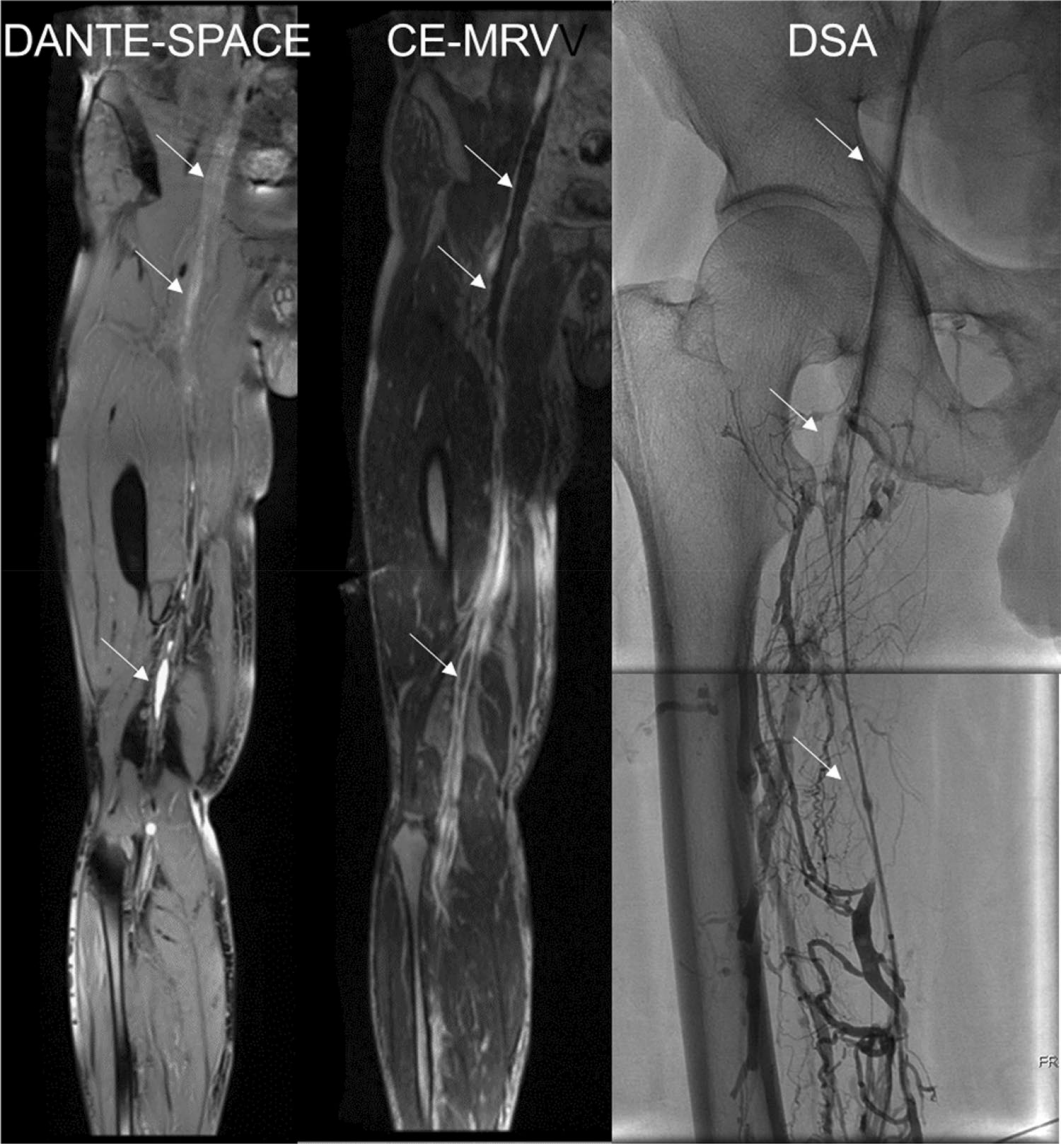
MRI and DSA images of a 61-year-old male with right lower extremity swelling and pain. In the DANTE-SPACE images, the venous thrombosis of the right lower extremity showed a heterogeneous high signal with a rich signal hierarchy and a rough vascular wall in the diseased segment. In the CE-MRV images, there were multiple thromboses in the right lower extremity veins. The thrombus showed a low signal “filling defect,” and the difference in the thrombus signal was not obvious. In the DSA images, there was a right lower extremity vein occlusion and collateral circulation formation. The right iliac and femoral vein proximal thrombosis was easy to dissolve, and contrast agent filled the cavity. The thrombosis in the middle femoral vein was not easy to dissolve; after the catheter was passed through the cavity, contrast agent still did not fill it
